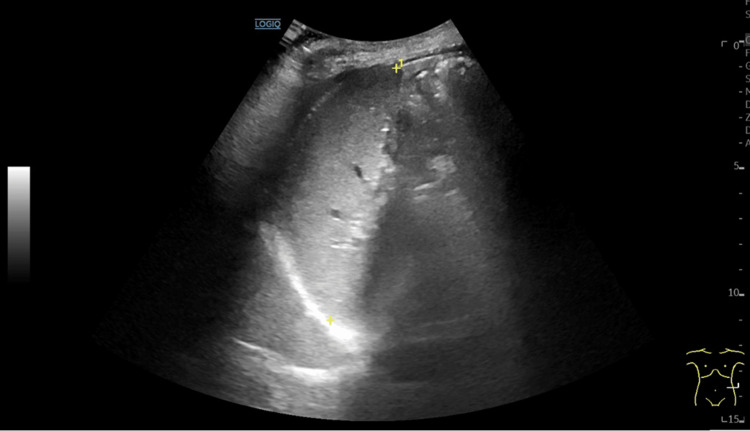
Abdominal ultrasound (B). The spleen had normal dimensions (10.3 cm), regular contours, and homogeneous parenchyma. The yellow asterisks represent the longitudinal length of the spleen.
